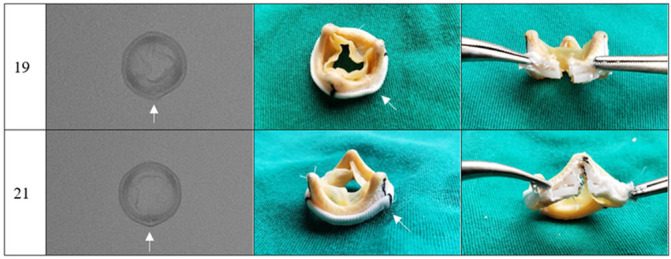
Labcor TLPB prosthesis after fracture, sizes 19 mm and 21mm Radiography (superior-inferior projection) and photographs (intact lining and dissected lining). Arrow corresponding to the fracture site.
